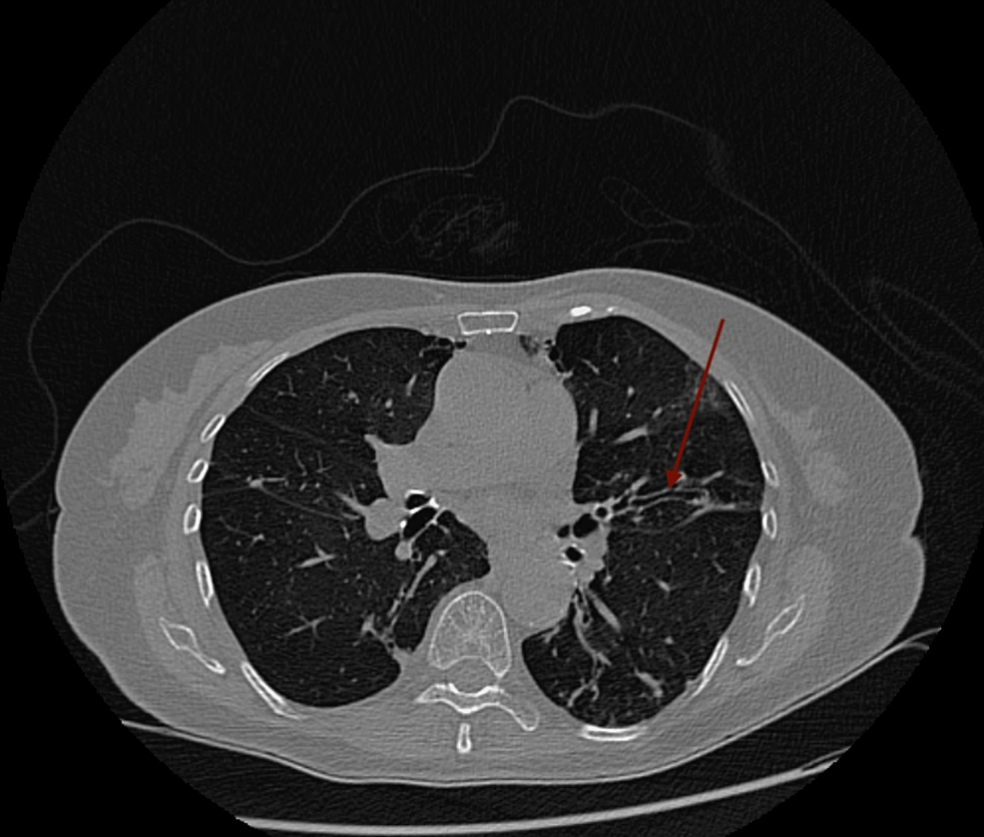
Cylindrical bronchiectasis in CT thorax with oral contrast.
